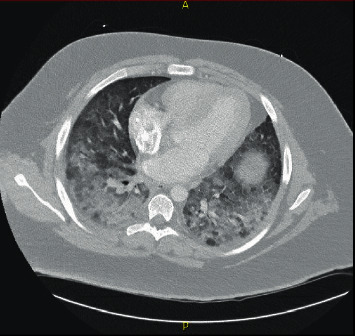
Chest CT angiography showing diffuse symmetric ground-glass opacities with confluent consolidation within both lower lobes.
